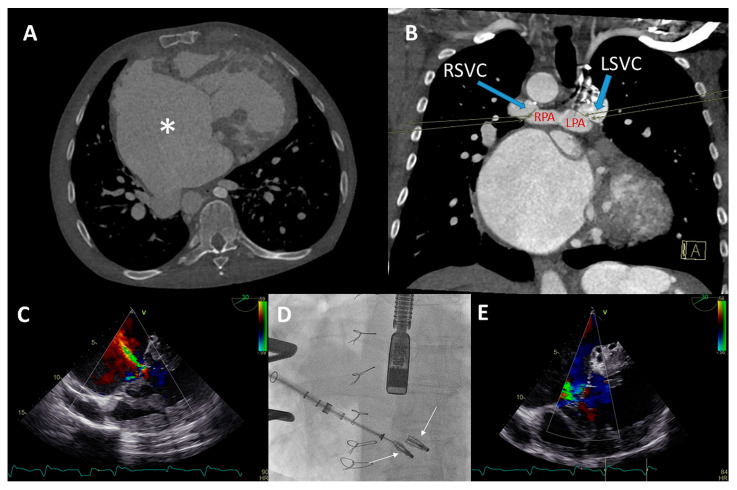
(A,B) Computed tomography (CT) scan of the thoracic cavity with the anatomical position of the dilated functional mono-atrium (asterisk) against the right thoracic wall (panel A) and bidirectional bilateral Glenn shunt (panel B) (LPA = left pulmonary artery, RPA = right pulmonary artery, LSVC = left superior vena cava, RSVC = right superior vena cava). (C) Pre-procedural transesophageal echocardiography (TEE) which visualized severe common atrioventricular (AV) valve regurgitation. (D) Anteroposterior fluoroscopic view of the two XTW MitraClips positioned in the AV valve. At the same time, it is appreciated that the delivery system is positioned through the fifth intercostal space after a right mini-lateral thoracotomy. (E) Moderate AV regurgitation after the hybrid procedure visualized with TEE.
